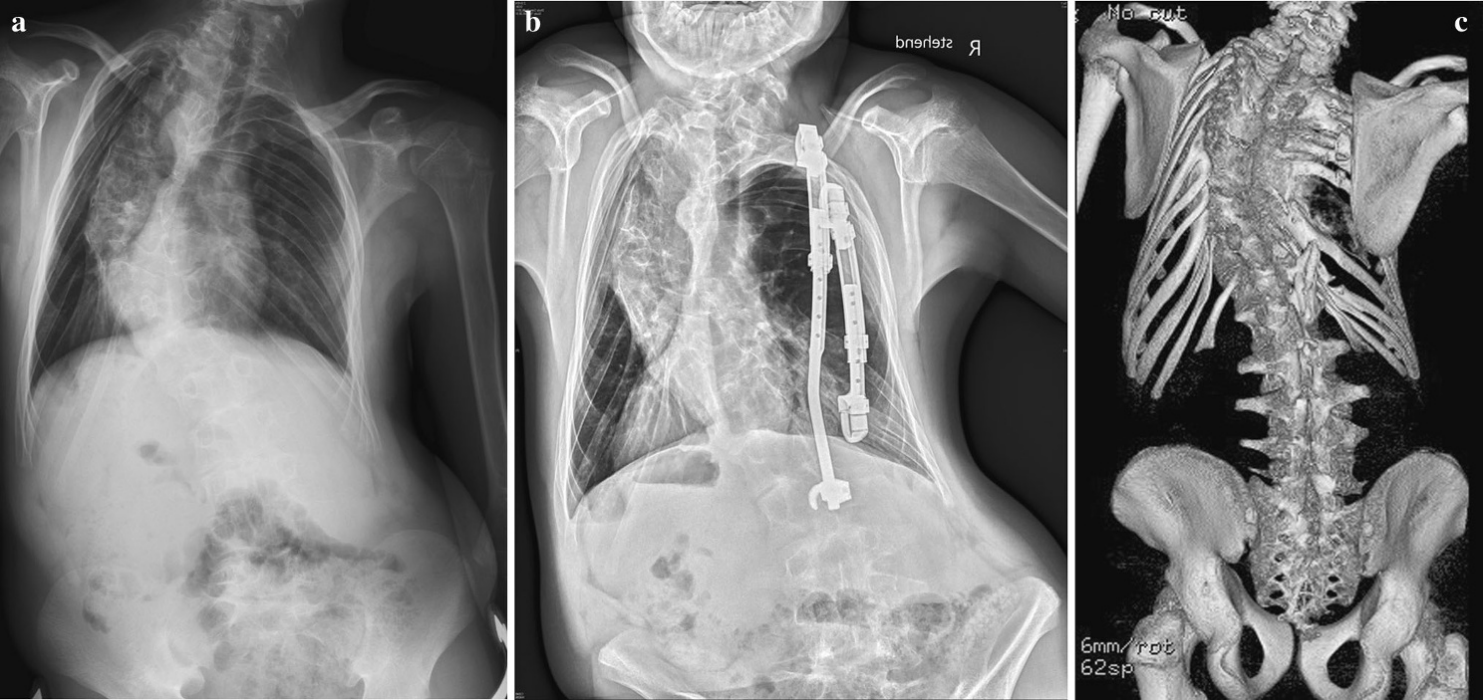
a Twelve-year-old boy with congenital scoliosis prior to the index procedure. b Four years later, new bone formation along the rod section of the inner VEPTR constructs was suspected. c A CT 7 years later (at the age of 19 years) confirmed the presence of ossifications at the level of the former lamina hook (Ib) reaching over the caudal ribs (IIa), and also at the level of the former upper rib cradle (Ia). The thoracostomy remained open even after removal of the VEPTR. Based on those findings, a decision was made not to instrument and fuse the spine, since the situation was deemed stable
